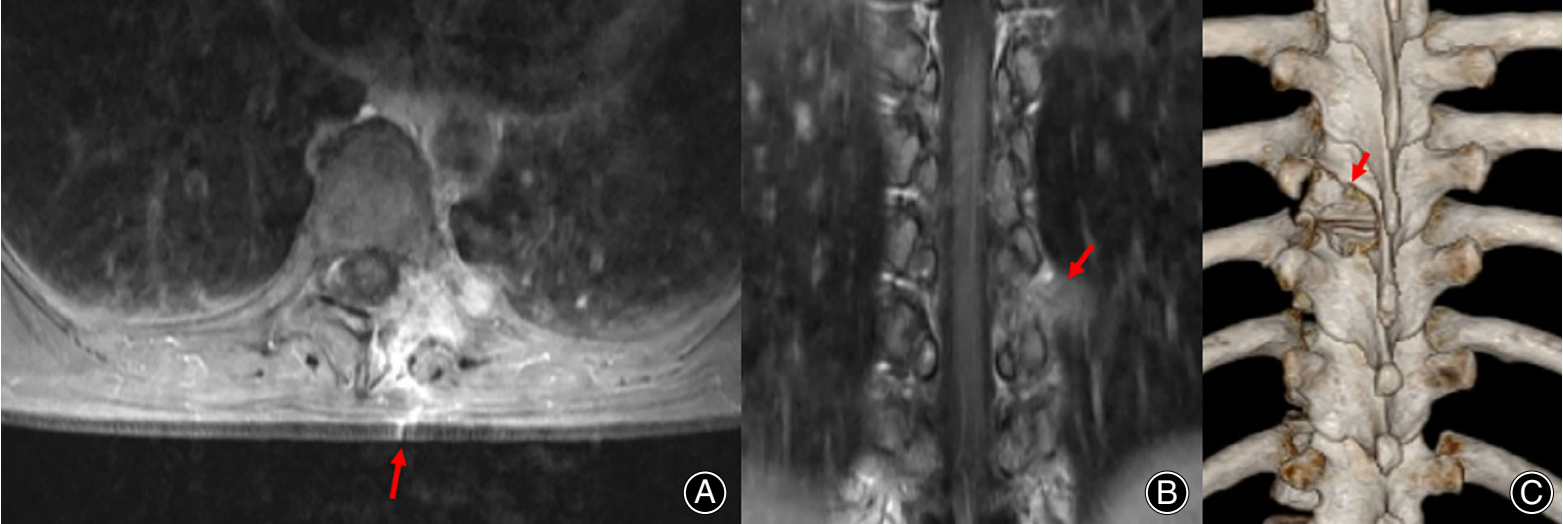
Postoperative T1‐weighted enhanced MRI on axial (A) and coronal (B) planes. Resection of the dumbbell tumor could not be identified (arrow) after 6 months. Postoperative 3D‐CT scan shows the resected vertebrae (C).
